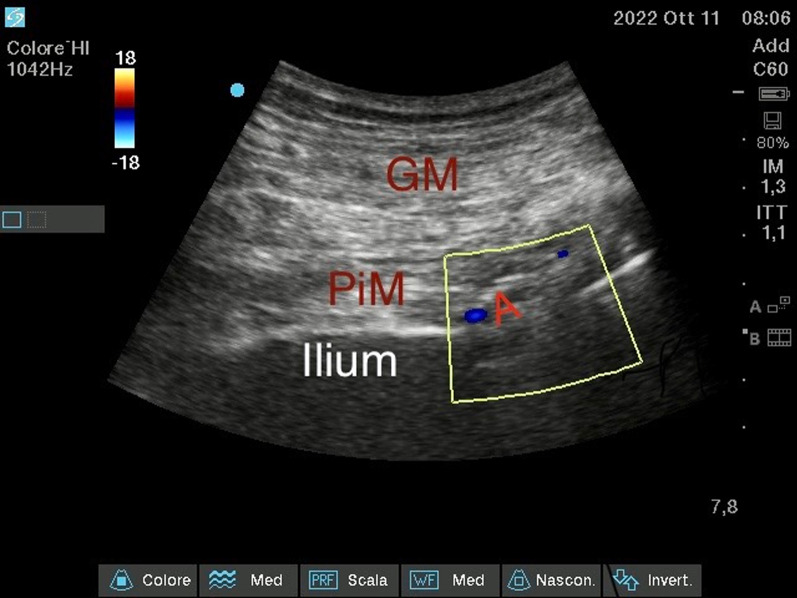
Ultrasound Sciatic Nerve block—Parasacral approach—ecocolordoppler image. Gluteal muscle (GM); Piriformis muscle (PiM); Gluteal artery (A)
